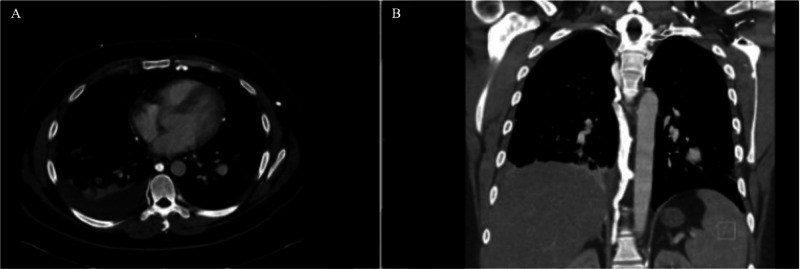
CTA Chest with pulmonary artery imaging with intravenous contrast. Axial (panel A) and coronal (panel B). A pulmonary artery aneurysm measuring 1.6 cm was identified in the left lower lobe. There is evidence of chronic, complete occlusion of the SVC, accompanied by the presence of extensive collateral veins observed in the chest wall, mediastinum, pericardium, and upper abdomen.
